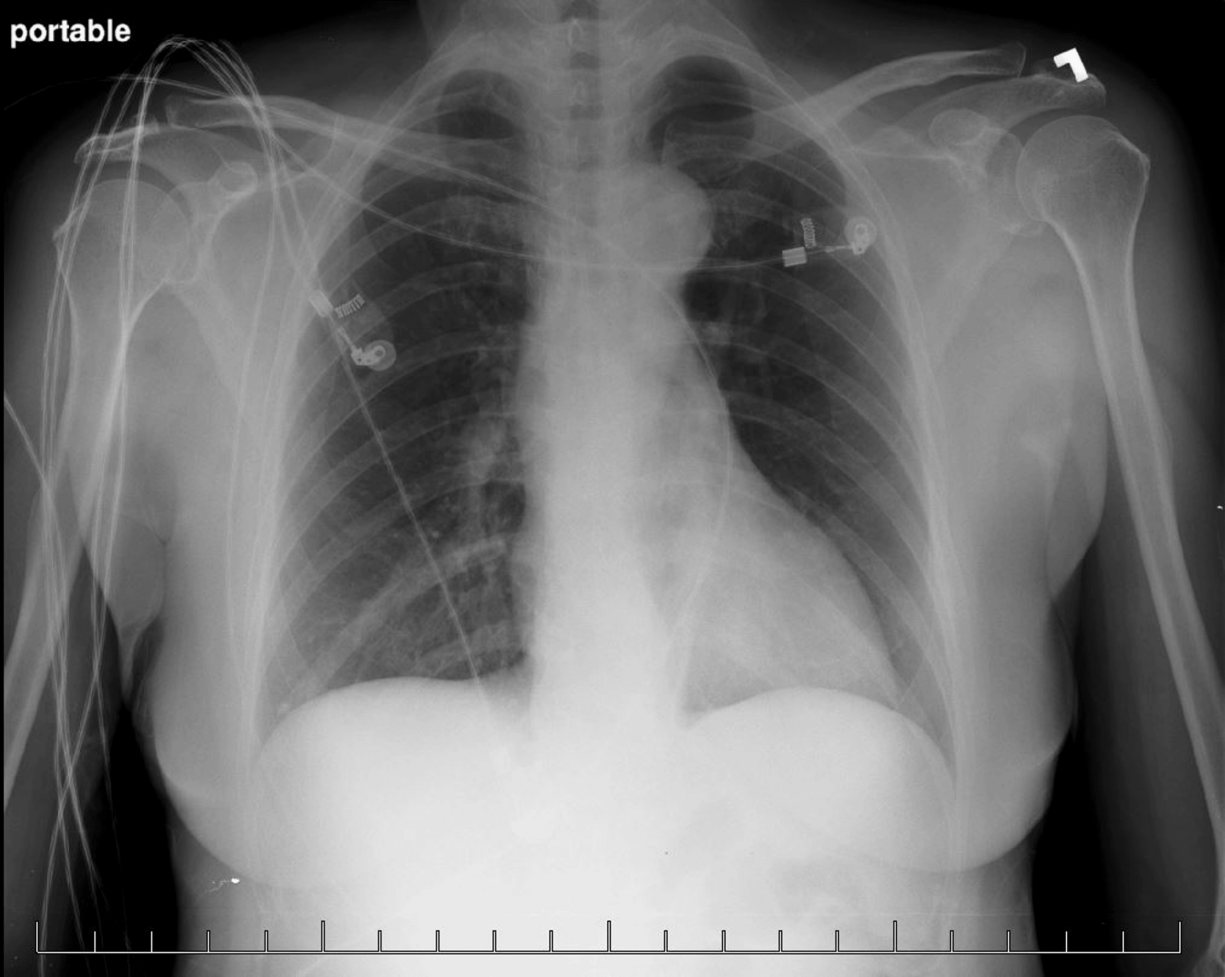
Chest X-ray in AP showed a possible infiltrate on the middle and lower lobes of the right lung.
